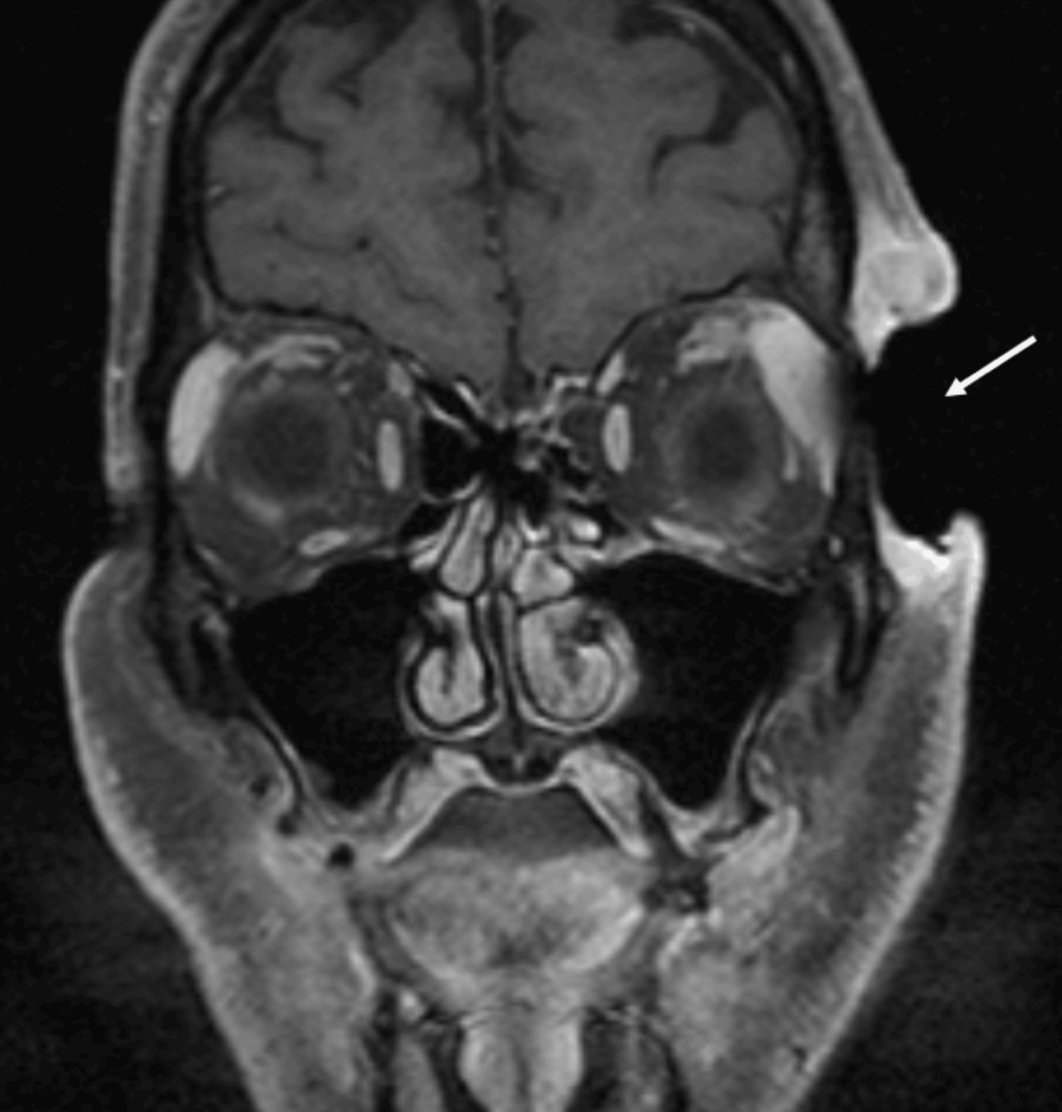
Coronal T1‑weighted post‑contrast fat‑suppressed MRI of the orbits demonstrating erosion of the left craniofacial soft tissues.
